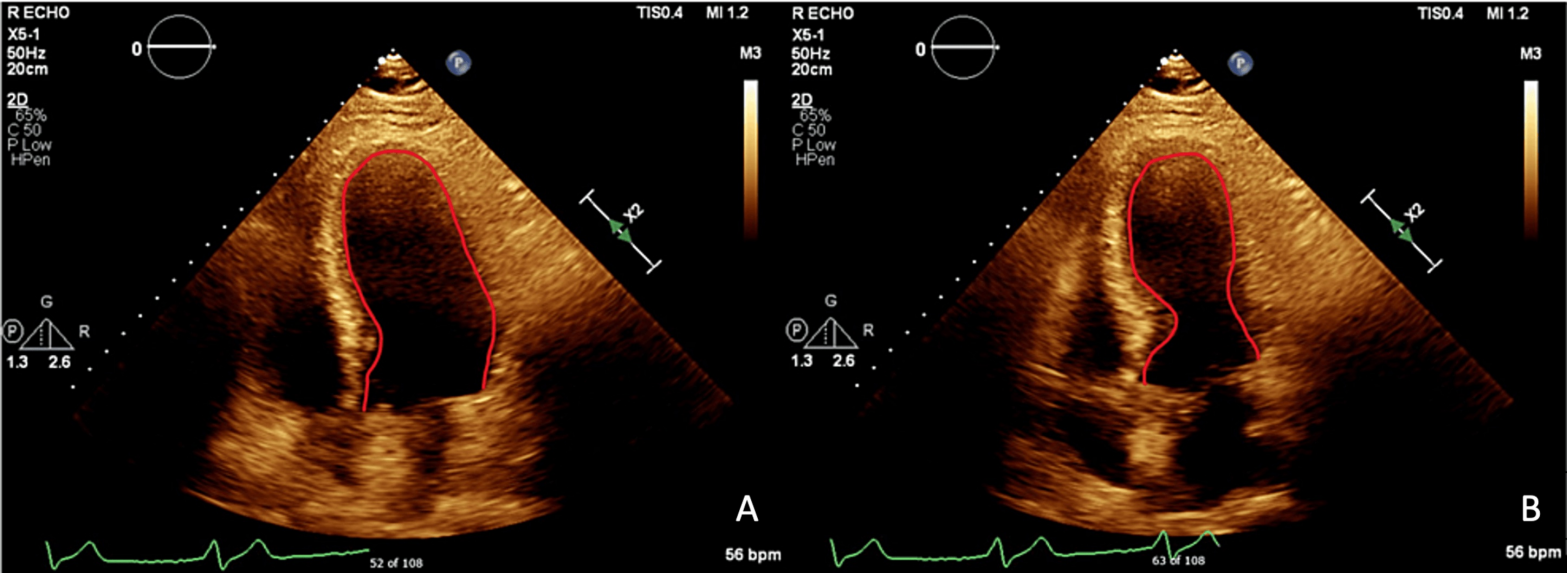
Apical four-chamber view on transthoracic echocardiogram with diastolic phase on the left (A) and systolic phase on the right (B). The red outline shows classic apical akinesis with basilar sparing and left ventricular dilatation.
